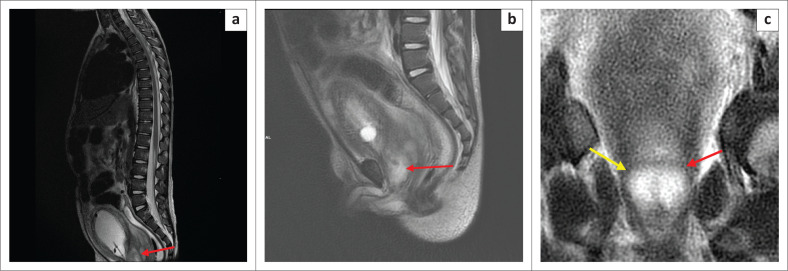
Magnetic resonance imaging: Sagittal T2-weighted sequence (a) of the thoracic and lumbar spine. Incidental cyst of the prostate gland was noted. Sagittal and coronal T2-weighted sequences (b and c, respectively) of the pelvis demonstrate a well-defined homogenous cystic lesion within the prostate gland in keeping with a prostatic cyst.
